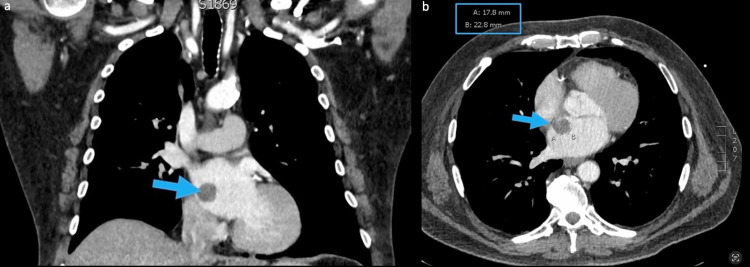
CTA in sagittal (a) and axial (b) views. Pedunculated 2.3 × 1.8 cm hypoattenuating left atrial mass arising from the fossa ovalis of the interatrial septum (arrows). CTA: computed tomography angiography
